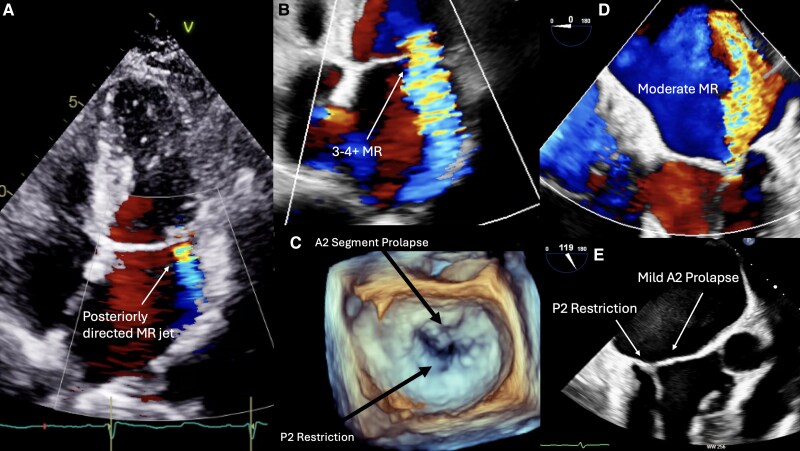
Echocardiographic images at various time points. (*A*) Baseline TTE in February 2024 showing posteriorly directed trivial MR in the Apical 4-chamber view. (*B*) Moderate to severe MR noted on July 2024 TTE in the apical 4-chamber view (*C*) 3D surgeon’s view of mitral valve with pseudo-prolapse of A2 segment due to significantly restricted P2 segment on TEE (*D*) Moderate posteriorly directed MR at 0° mid-oesophageal view on TEE. (*E*) 120° mid-oesophageal view of the mitral valve of TEE again showing pseudo-prolapse of the A2 segment and restriction of the P2 segment.
